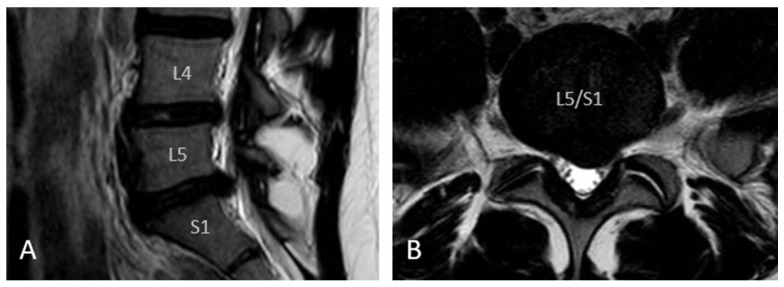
Sagittal (A) and axial (B) T2-weighted MRI of an adolescent patient showing a left broad-based L5/S1 disc herniation. Informed consent was obtained from the patient for publication of this image.
